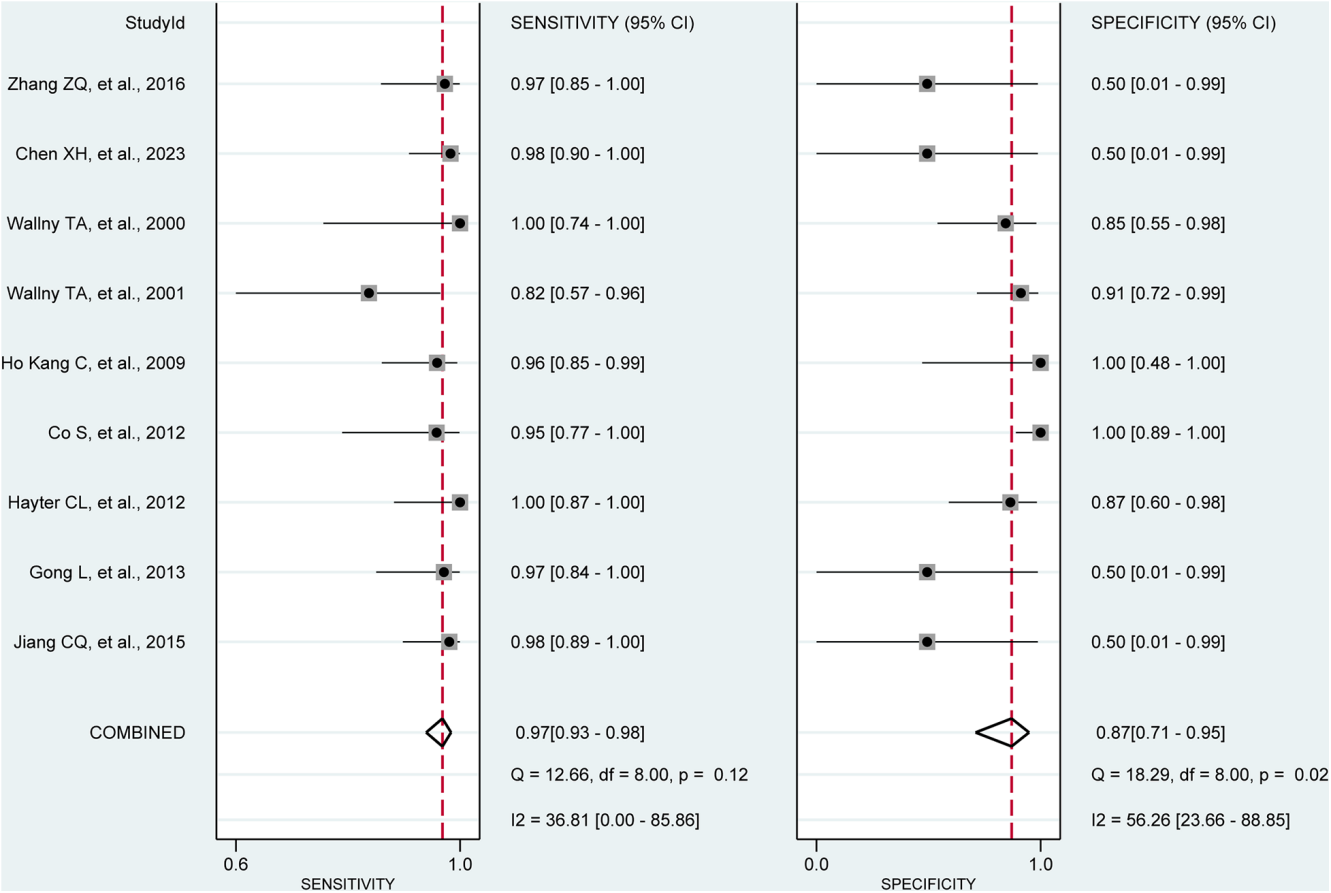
Forest plots of the pooled sensitivity and specificity to diagnose any rotator cuff tear with the corresponding 95% confidence region. Three-dimensional sonography for the diagnosis of patients with any rotator cuff tear (nine studies, the pooled sensitivity = 0.97, *I*^2 ^= 36.81%; the pooled specificity = 0.87, *I*^2 ^= 56.26%). CI, confidence interval.
